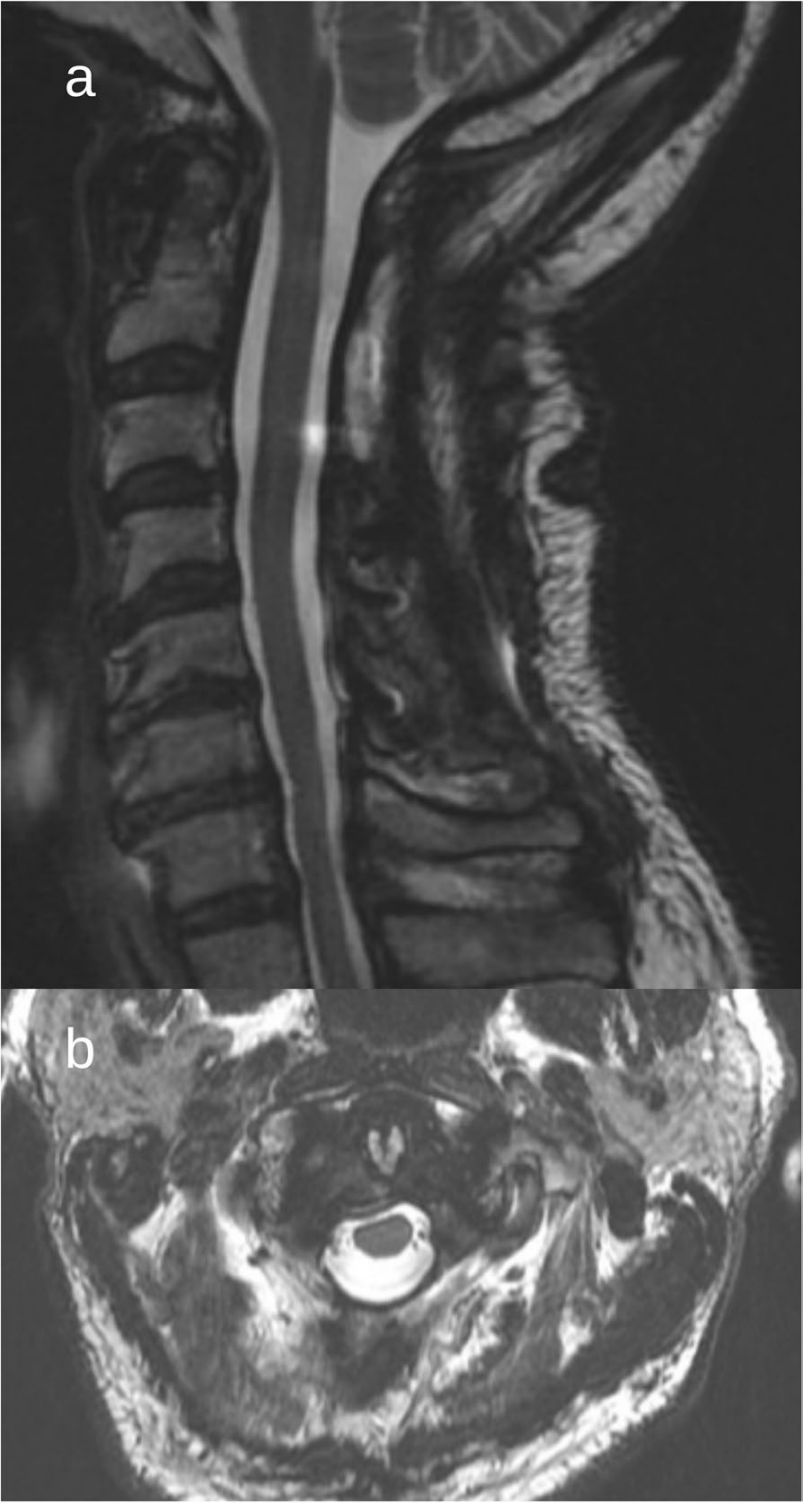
Postoperative MRI T2-weighted imaging: sagittal (a) and axial (b) views suggested substantial spinal cord decompression at C1 and C2
